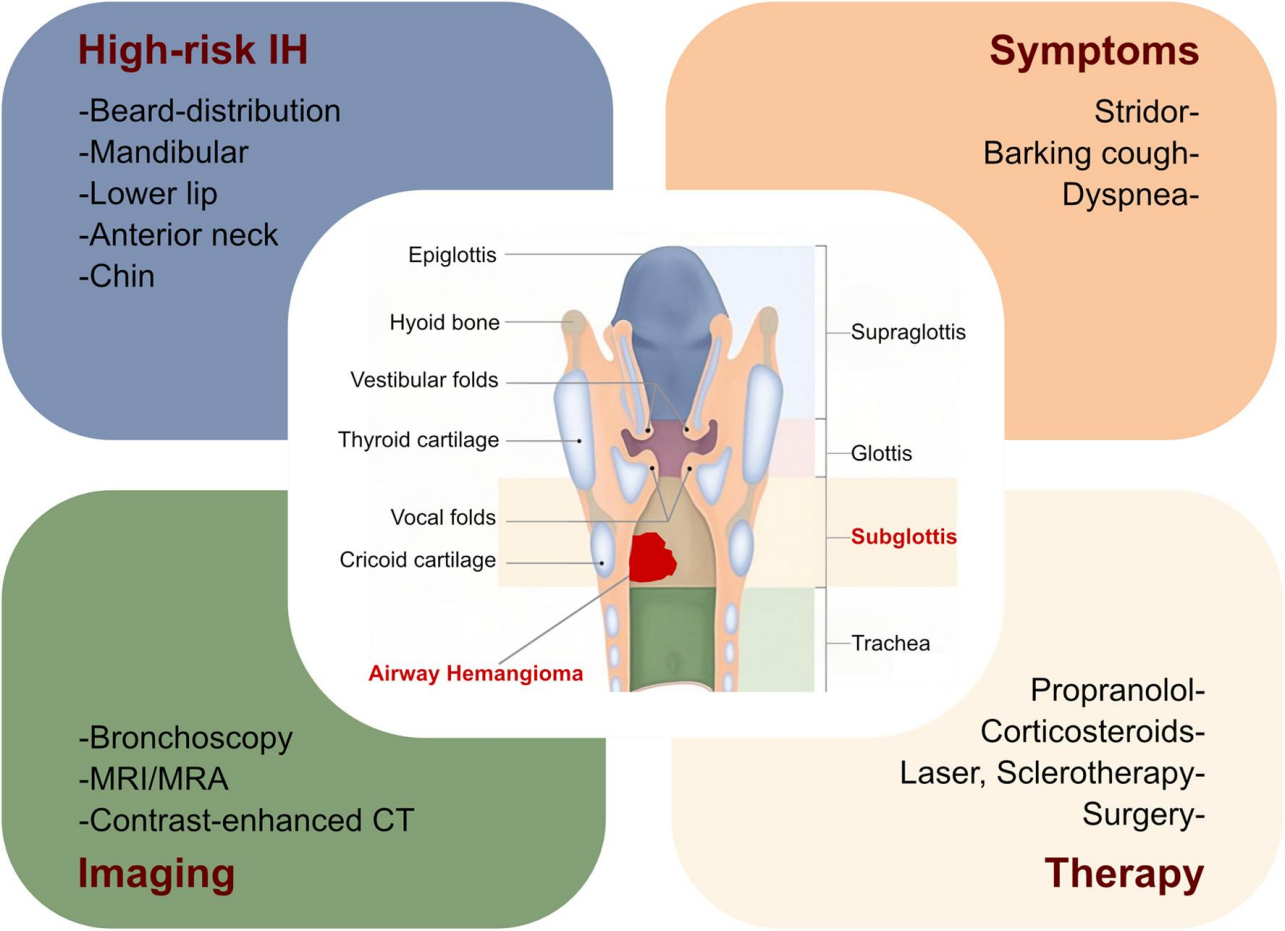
Summary of airway infantile hemangioma. Herein, we summarize the risk of obstructive airway hemangioma in patients with IHs, along with their clinical symptoms, imaging examinations, and therapies. MRI, magnetic resonance imaging; MRA, magnetic resonance angiography; CT, computed tomography; IH, infantile hemangioma.
